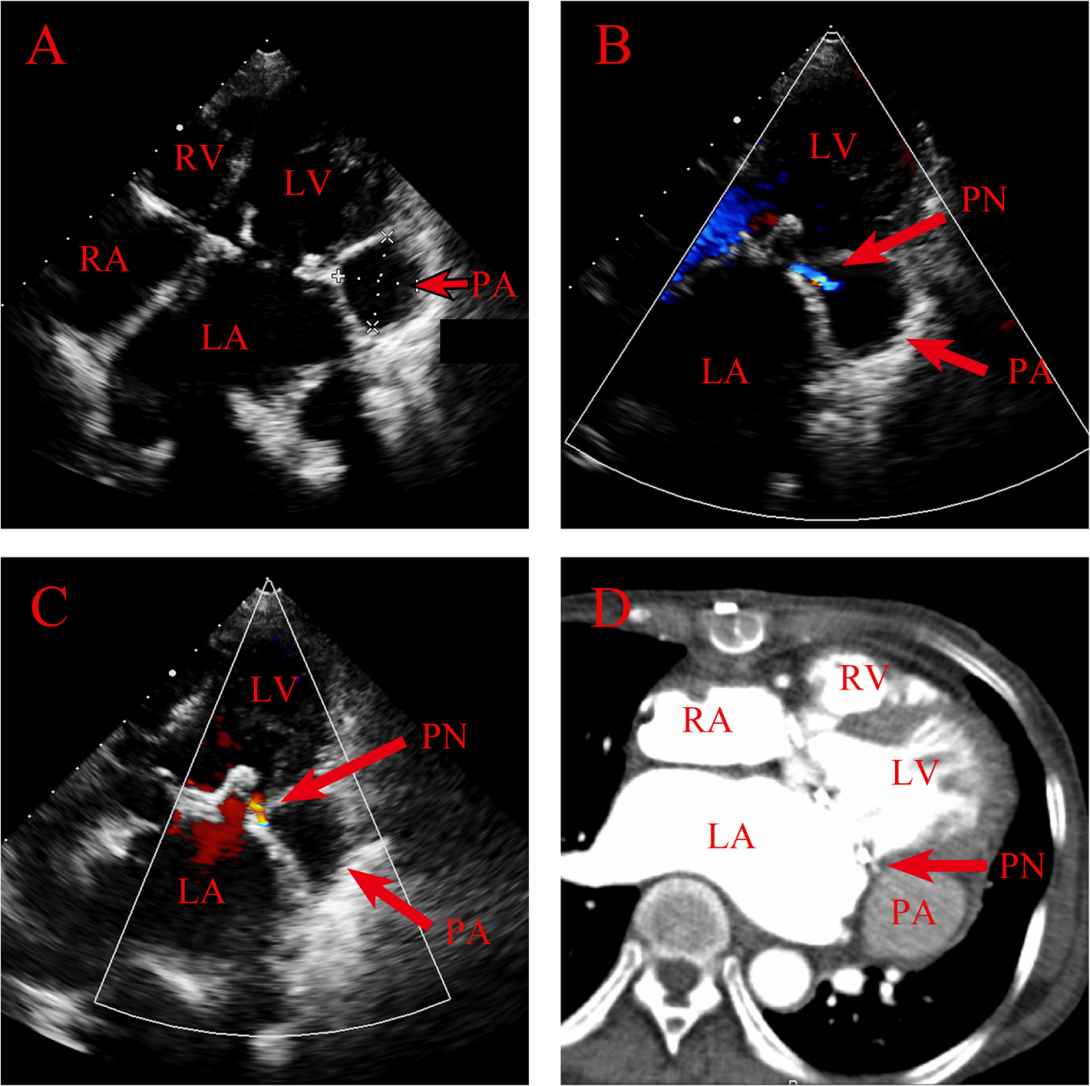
a Two-dimensional transthoracic echocardiogram in apical four-chamber view showed an aneurysm-like structure measuring (29 × 25 mm) suggestive of a submitral aneurysm. Color Doppler imaging revealed blood inflow from LV to pseudoaneurysm (b) and from pseudoaneurysm to LV (c) through a narrow neck (measuring 3 mm) in systole. d Contrast-enhanced cardiac computed tomography confirmed the pseudoaneurysm. RV: right ventricle. RA: right atrium. LV: left ventricle. LA: left atrium. PA: pseudoaneurysm. PN: pseudoaneurysm neck
